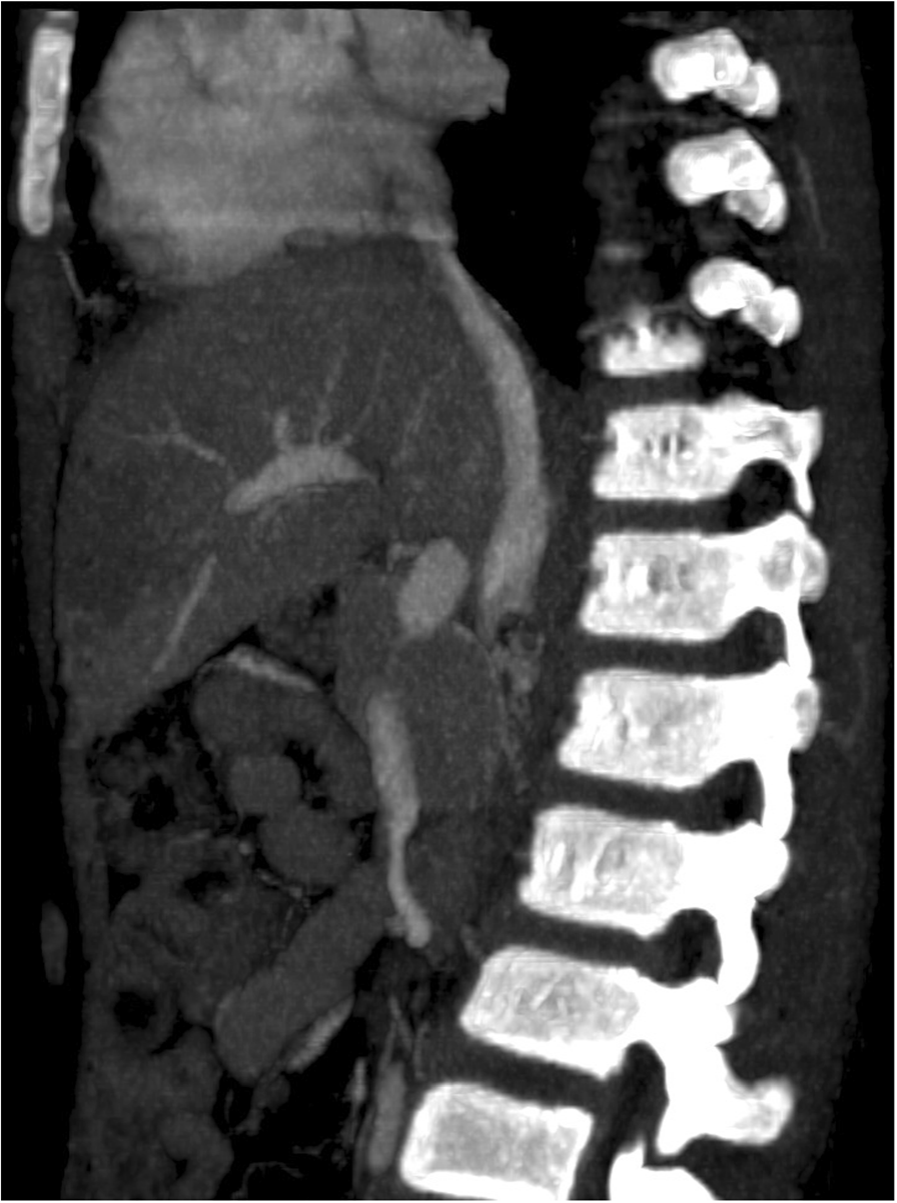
Computed tomography venogram (sagittal section) showing complete absence of infrarenal inferior vena cava and normal drainage of hepatic inferior vena cava into right atrium of her heart
